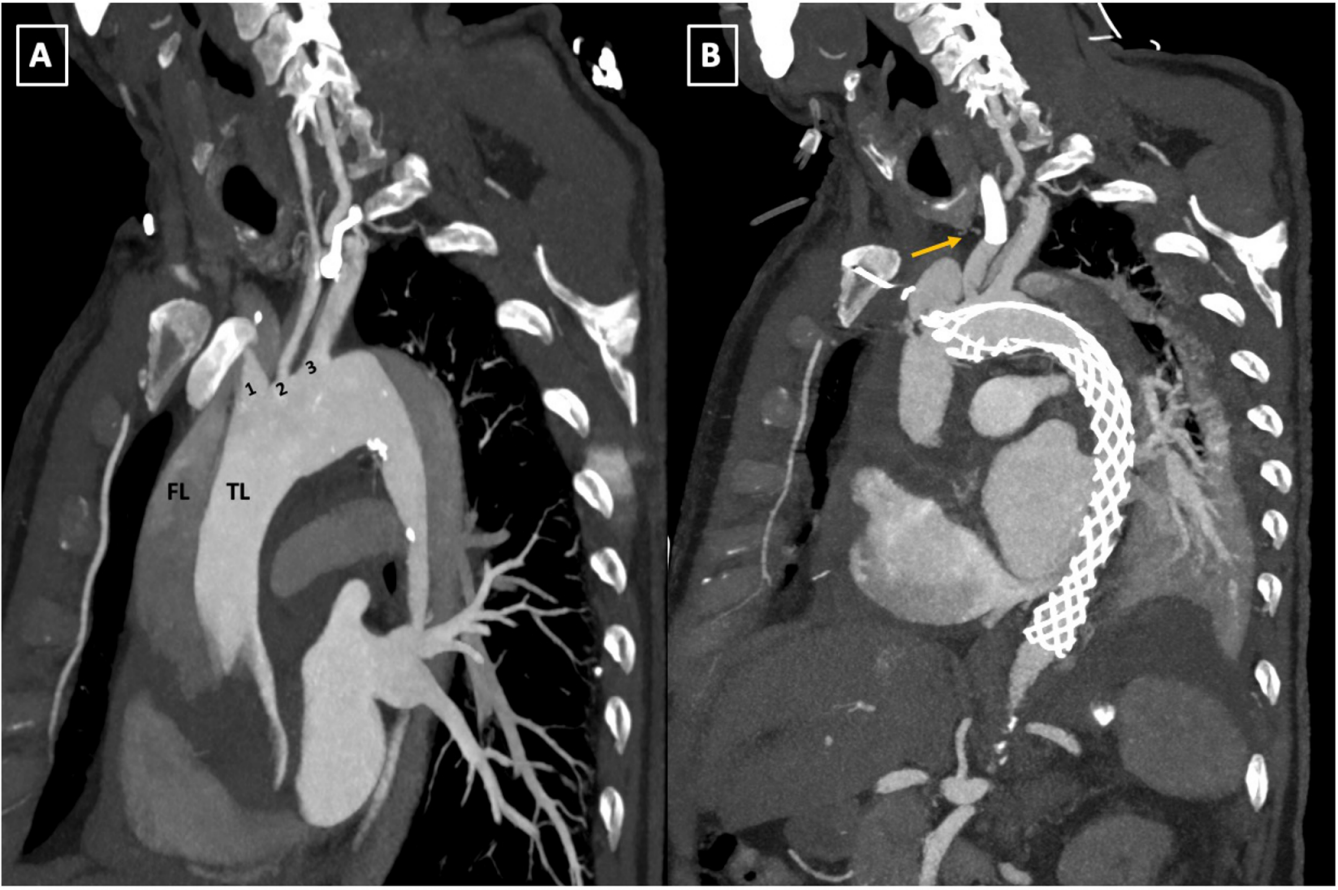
(A,B) Pre-and postoperative follow-up computed tomography angiography (CTA) scan comparison. The parasagittal reconstruction view of the aortic arch demonstrates changes placement of the AMDS stent in zone 0 of the aortic arch and left common carotid artery (LCCA) endovascular stent deployment (arrow, B). TL, true lumen; FL, false lumen; 1, innominate artery; 2, left common carotid artery; 3, left subclavian artery; AMDS, ascyrus medical dissection stent.
